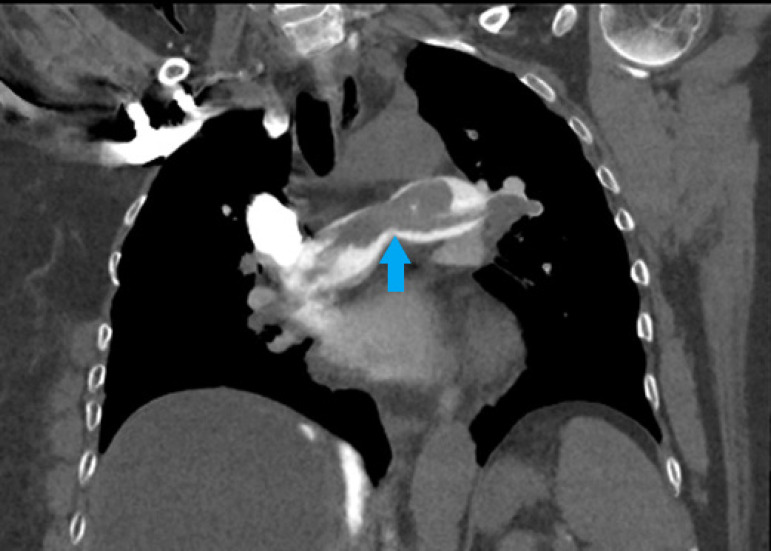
Computed tomography of pulmonary artery (coronal view). Arrow shows
